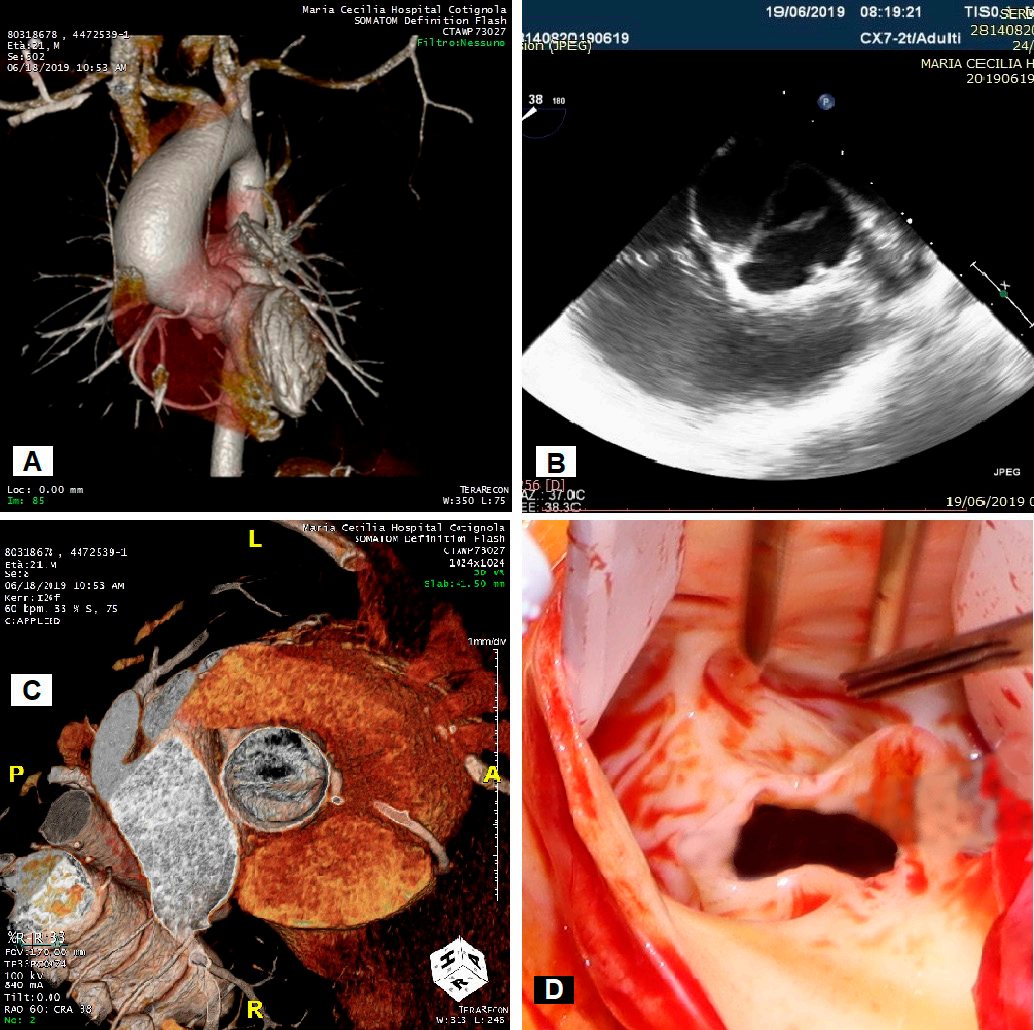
Pre- and intra-operative imaging. (A) Chest CT scan with 3D reconstruction - General view of the heart and aorta before repair. (B) Preoperative trans-oesophageal echocardiogram. (C) Chest CT scan with 3D reconstruction - Planar view of the unicuspid aortic valve. (D) Surgical view of the unicuspid aortic valve.
